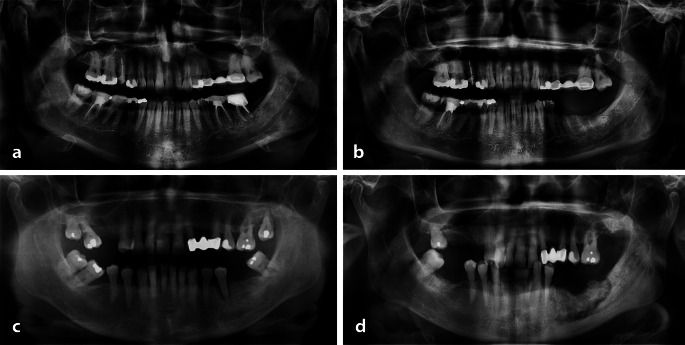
Panoramic radiograph of control and polypharmacy patients following multiple tooth extractions. Control patient: (a) pre-extraction image of teeth 36 and 37; (b) normal healing of alveolar socket at extraction sites of teeth 36 and 37. Polypharmacy patient: (c) pre-extraction of teeth 17, 28, 37, 35, 31, 41, 42, 47; (d) MRONJ observed at extraction sites of teeth 31, 41, 35, 37 and normal extraction site healing at 17, 28, 42, 47
